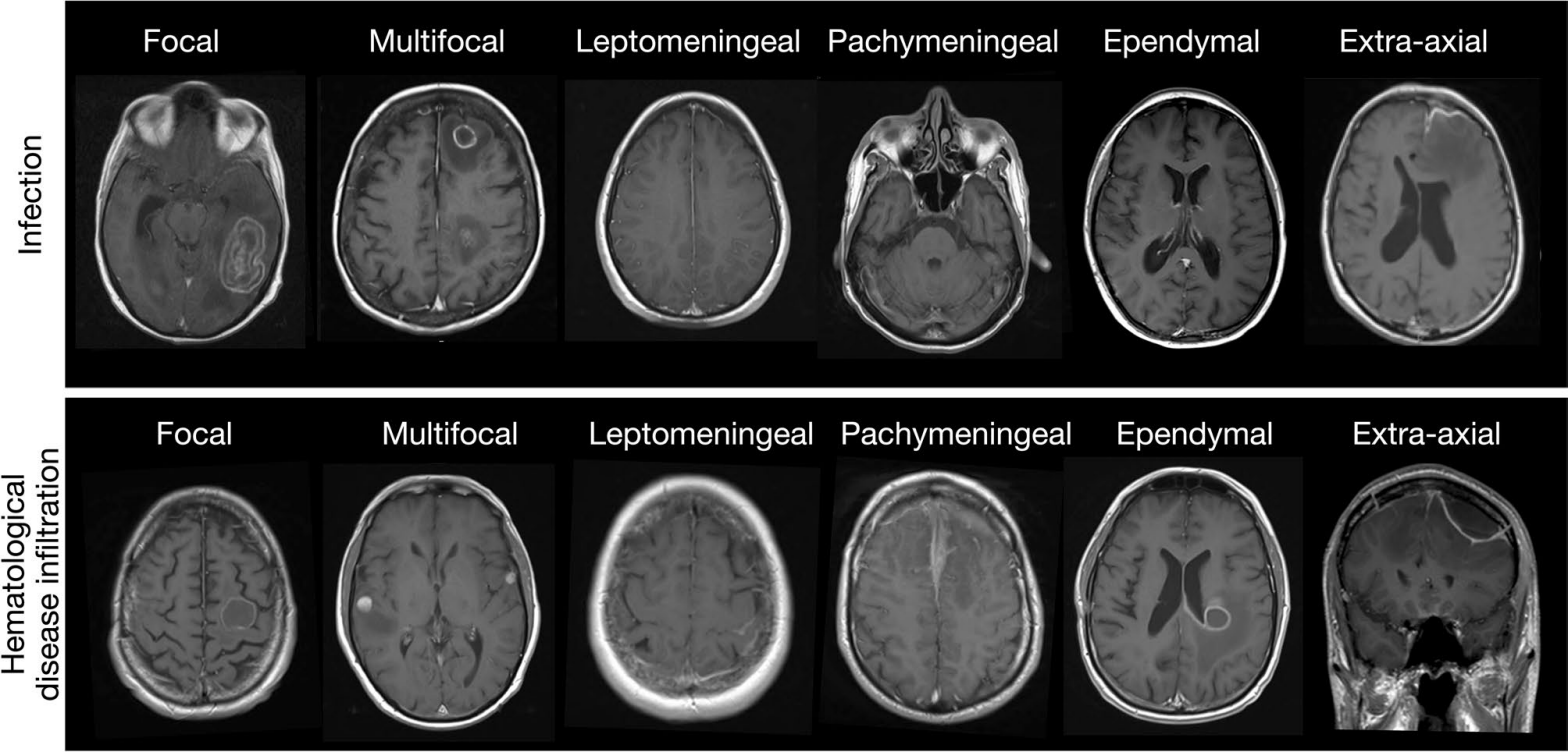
Case examples of anatomical imaging classification in MRI head studies with confirmed final diagnosis of either CNS infection of hematological disease involvement.
